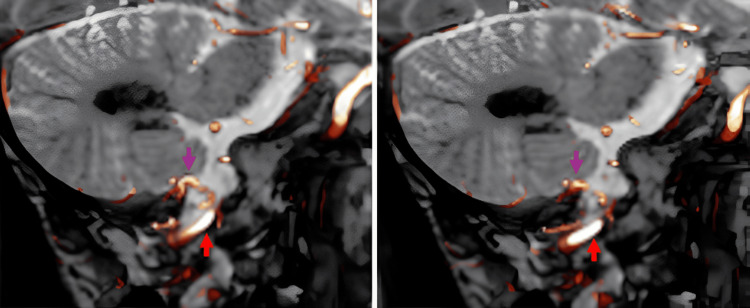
Sagittal fused images of CISS MRI, right VAG, and CCAG revealing a focal caliber change (purple arrow) at the suspected shunt point CCAG, common carotid artery angiography; CISS MRI, constructive interference in steady state MRI; VAG, vertebral artery angiography
